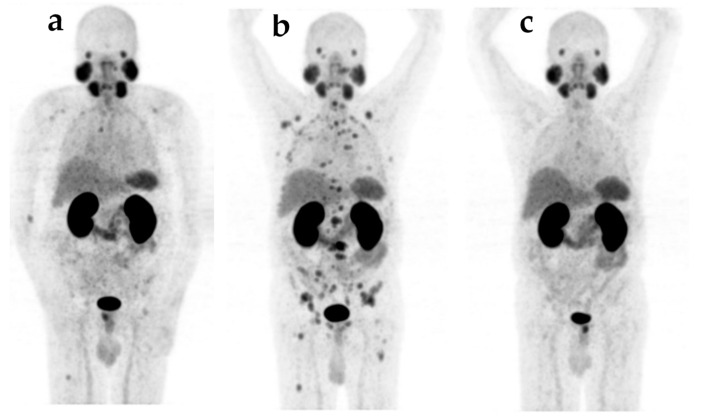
Maximum intensity projections of ^68^Ga-PSMA-11 PET/CT imaging in a 72-year-old patient (a) before ^223^Ra, (b) PD at baseline after ^223^Ra failure and (c) PR after 3 cycles of ^177^Lu-PSMA-617 and 25.3 GBq of cumulative treatment activity.
